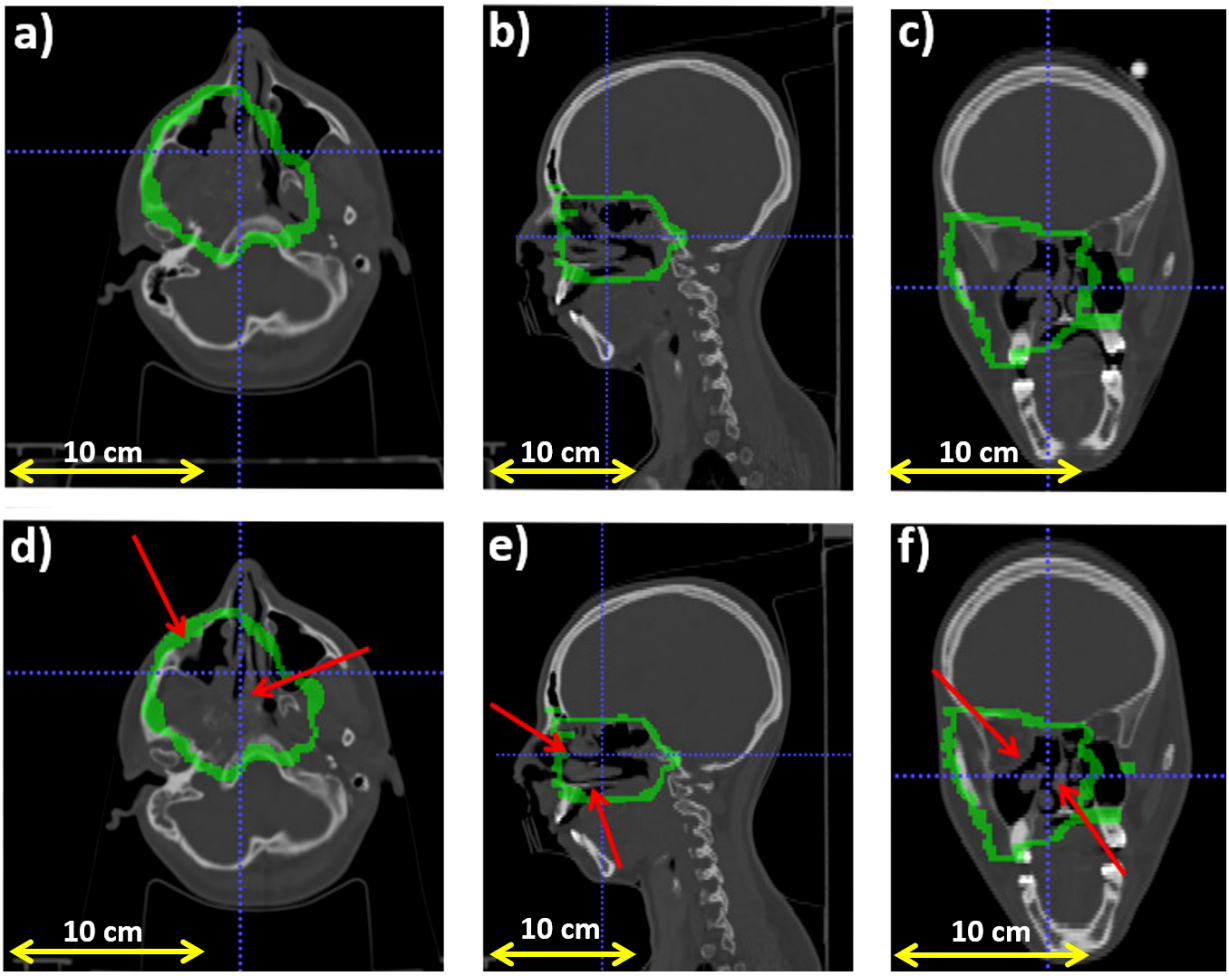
Planning CT (upper row) and control CT (lower row) for patient 006P. (A) Axial, (B) sagittal and (C) coronal views for the planning CT, and (D) Axial, (E) sagittal and (F) coronal views for the control CT. The morphological change is highlighted with a red arrow. Also, the CTV (Clinical Target Volume) area is highlighted with a green line.
